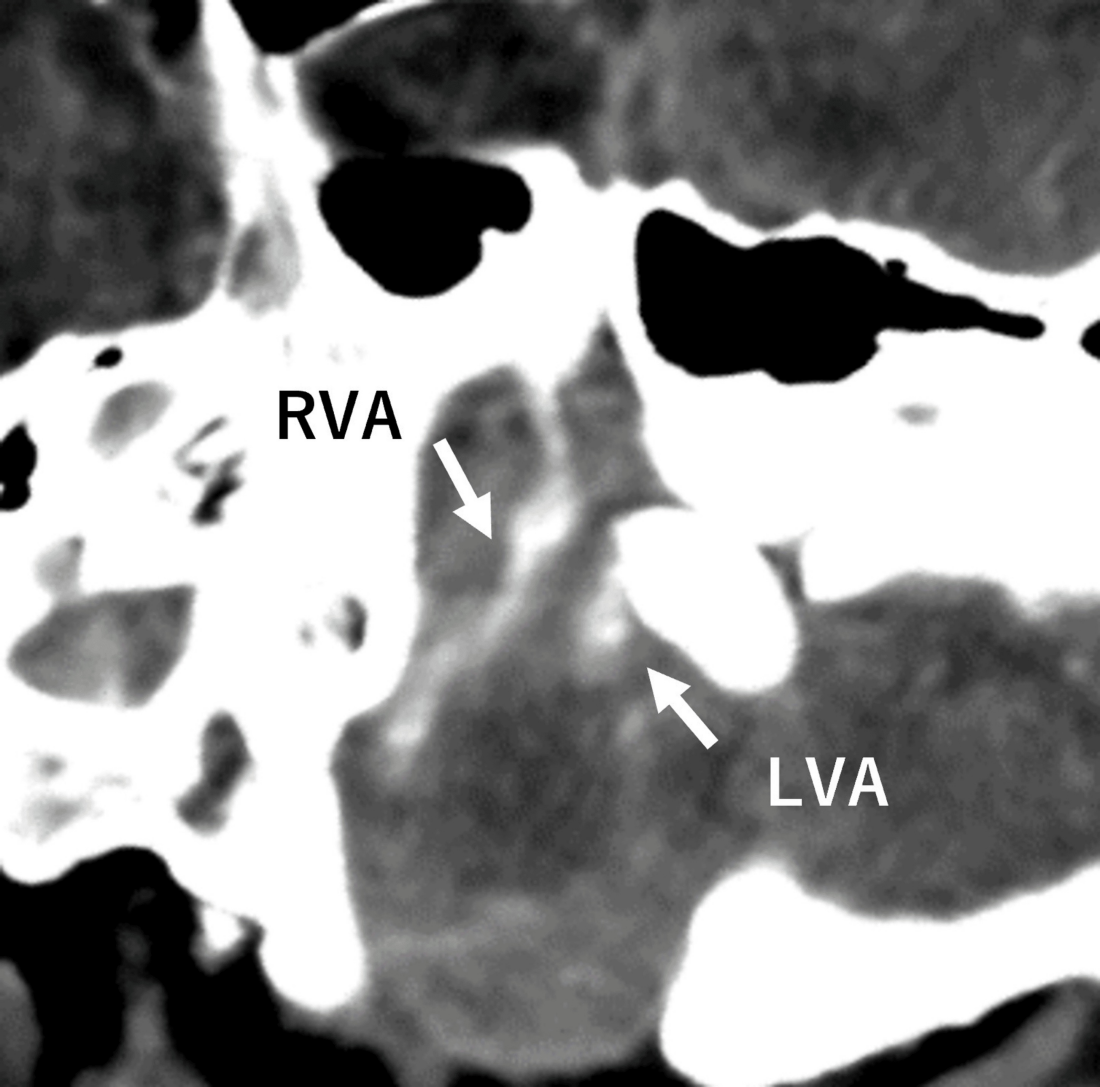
Computed tomography (CT) angiography performed following the non-contrast head CT reveals a ruptured aneurysm in the right vertebral artery, identified as the source of the subarachnoid hemorrhage. LVA: left vertebral artery; RVA: right vertebral artery
